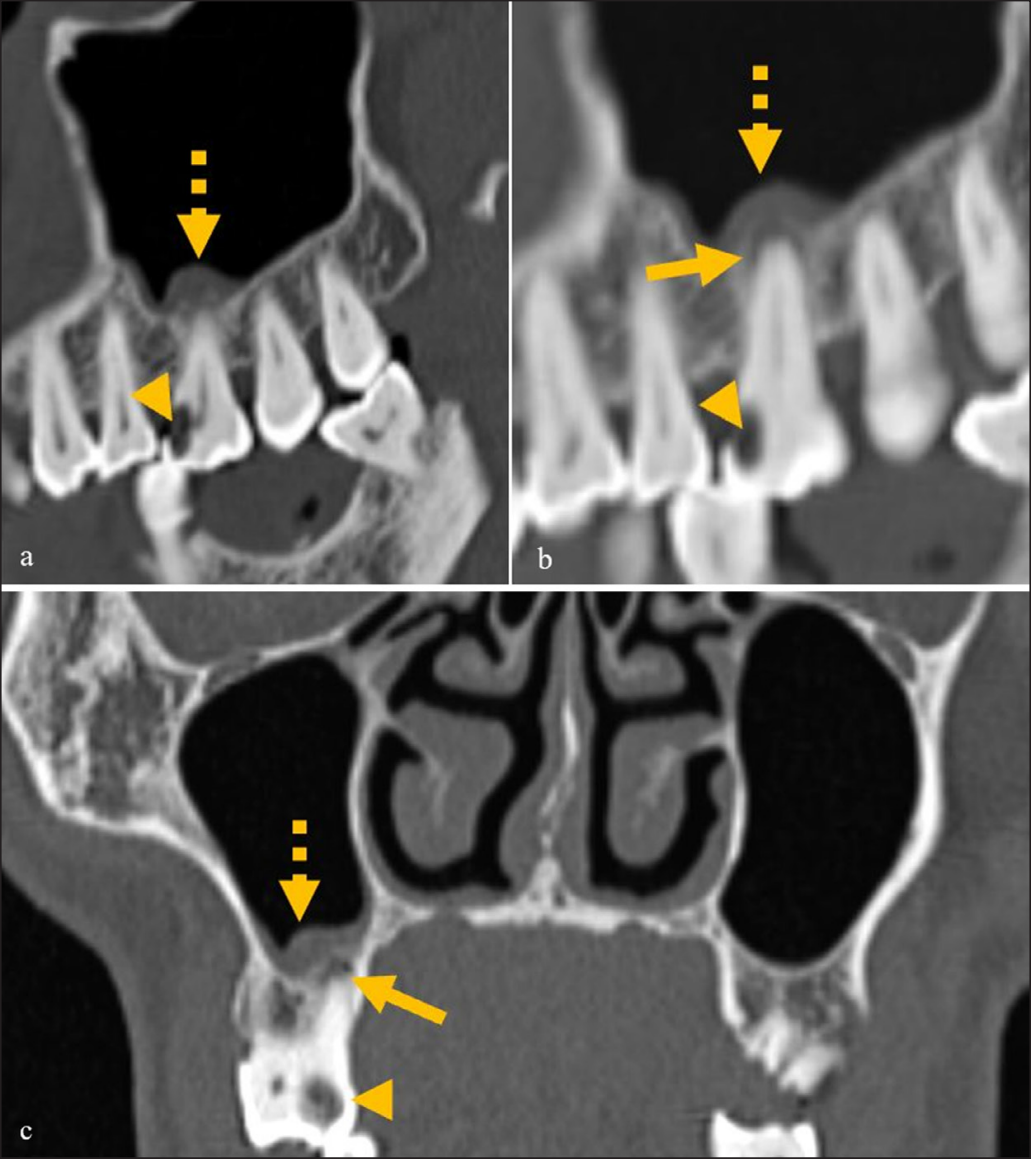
(a–c) A case of mild odontogenic sinusitis, showing dental caries (arrowhead) and mild periapical lucency (arrow) in the same tooth with mild mucosal thickening only adjacent to the diseased tooth (dashed arrow).
